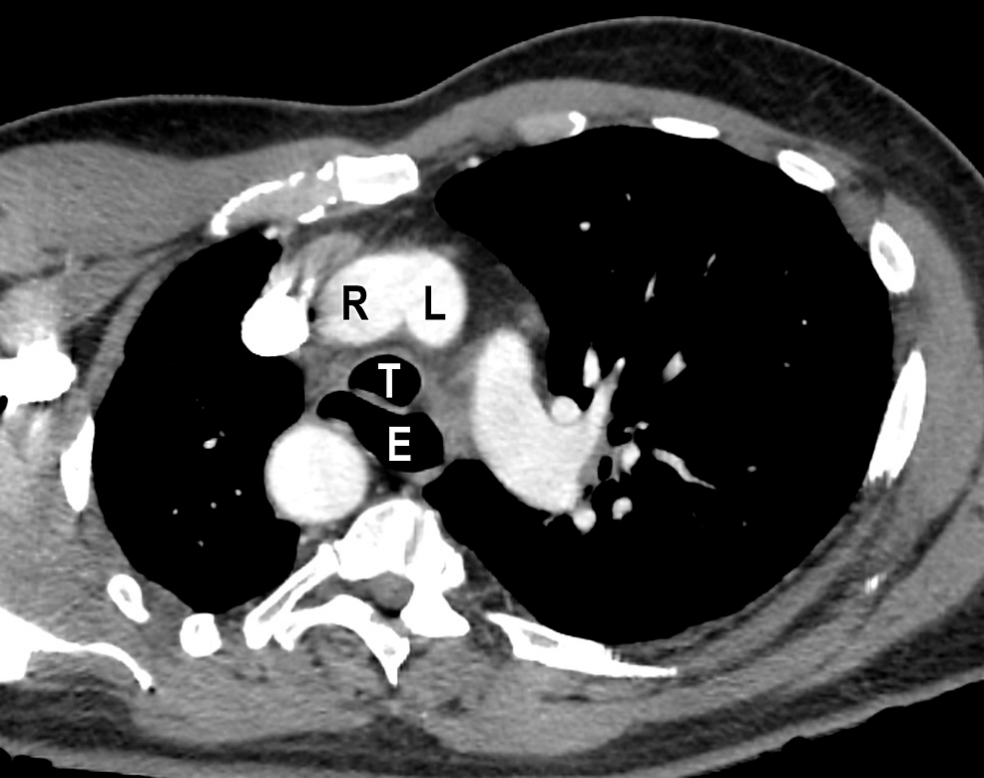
Oblique axial reformatted computed tomography image shows the ascending aorta splitting into the right (R) and left (L) limbs of the double arch anterior to the trachea (T). Note that the esophagus (E) is mildly distended at this level.
